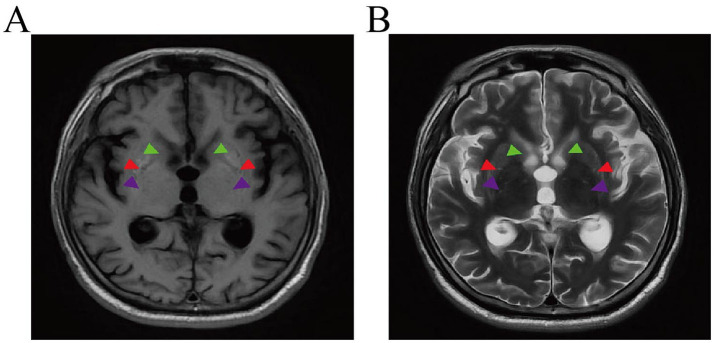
Abnormal signals in the bilateral caudate nucleus, putamen, and thalamus on T1-weighted (A) and T2-weighted (B) sequences. Green arrow: caudate nucleus; red arrow: putamen; purple arrow: thalamus.
